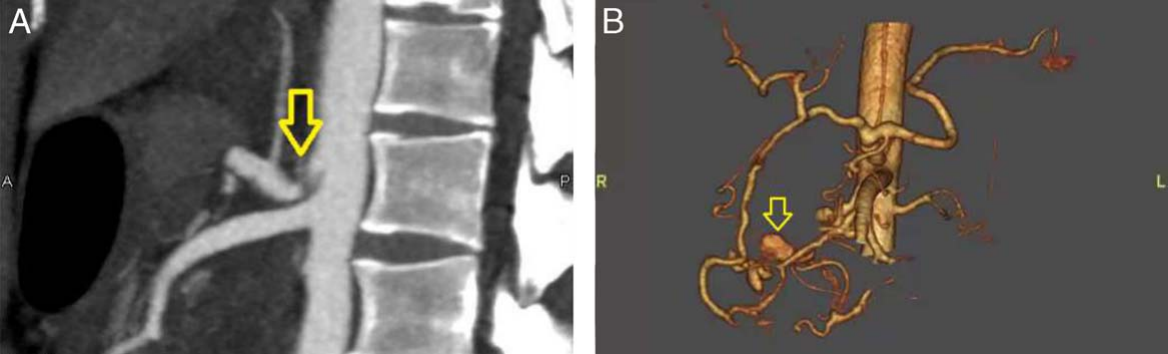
Pancreatoduodenal artery aneurysm due to median arcuate ligament syndrome. Contrast-enhanced computed tomography was performed. Blood flow from the superior mesenteric artery increases with compression of the celiac artery due to median arcuate ligament syndrome (A). This causes a change in hemodynamics within the pancreaticoduodenal arcade and forms an aneurysm (B).
